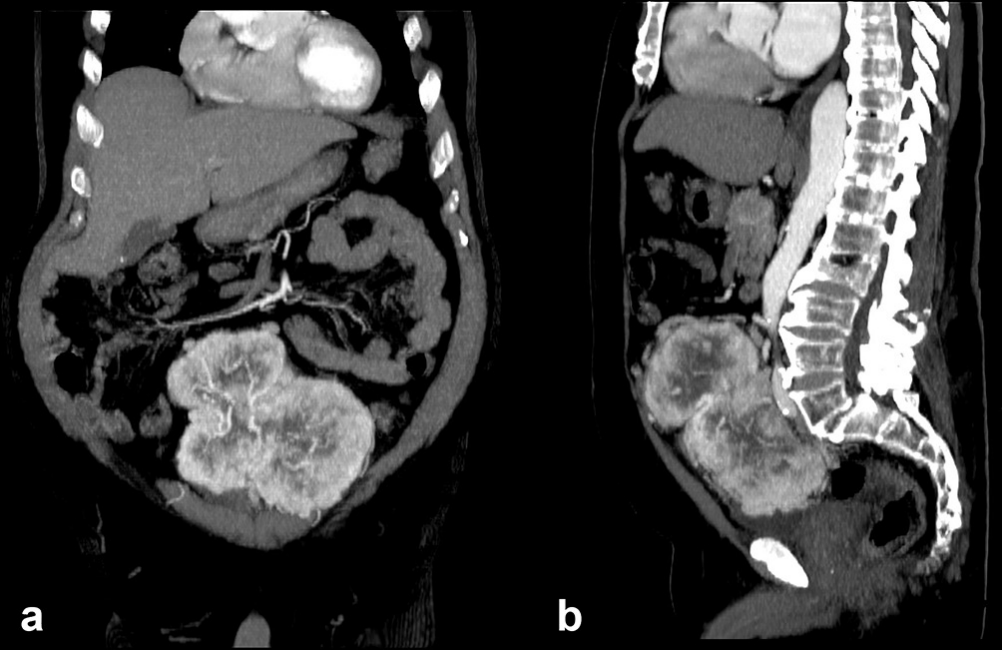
Enhanced arterial-phase CT demonstrates a multilobulated, well-defined, highly vascularized large mass situated at the mesentery with several areas of hypodensity corresponding with central necrosis.
